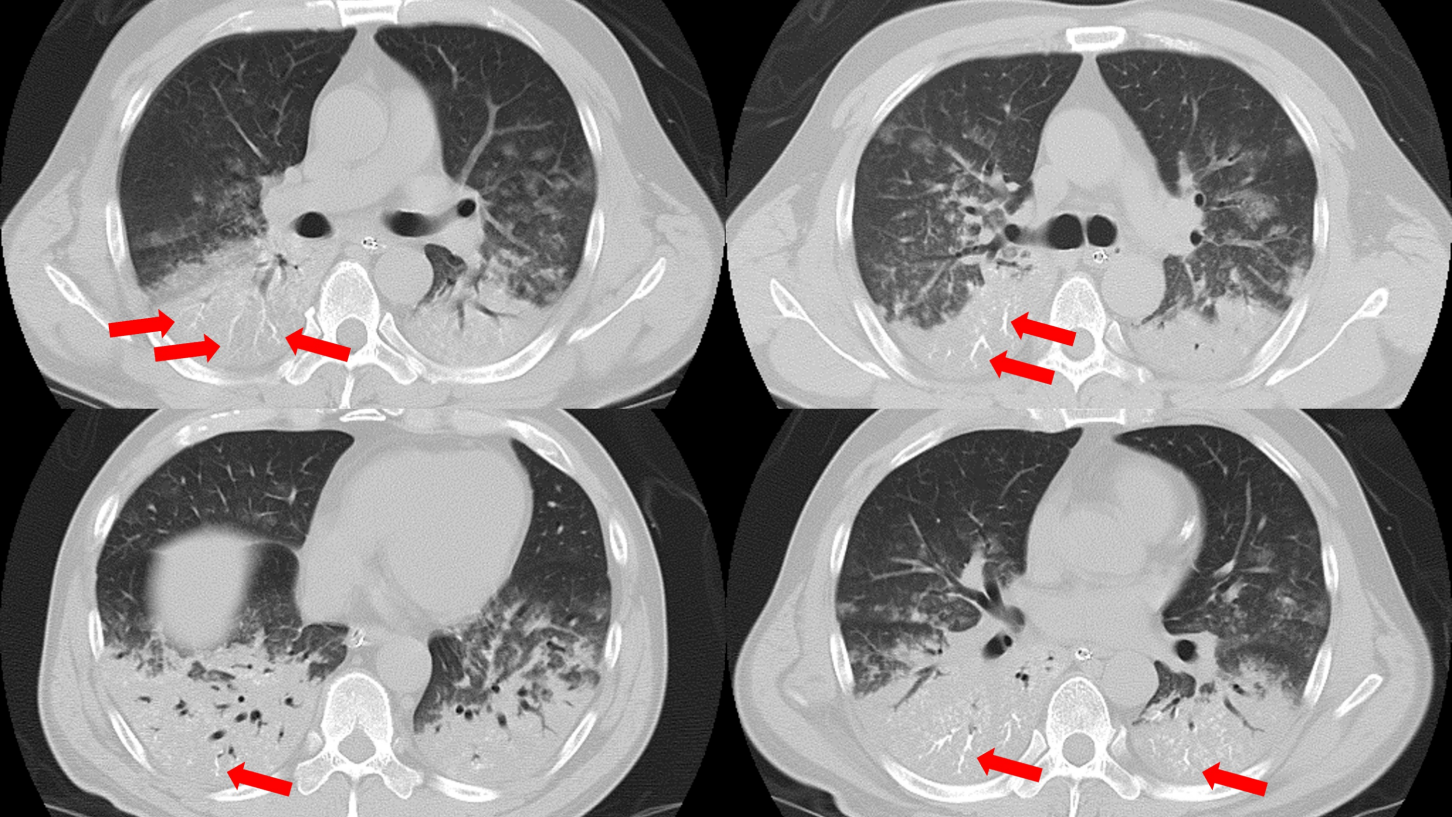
Axial chest computed tomography image on admission Extensive infiltrative shadows were observed in the bilateral dorsal lungs. In addition, there was a high-density finding of gravel and mud in the bronchi of the same area (red arrow).
